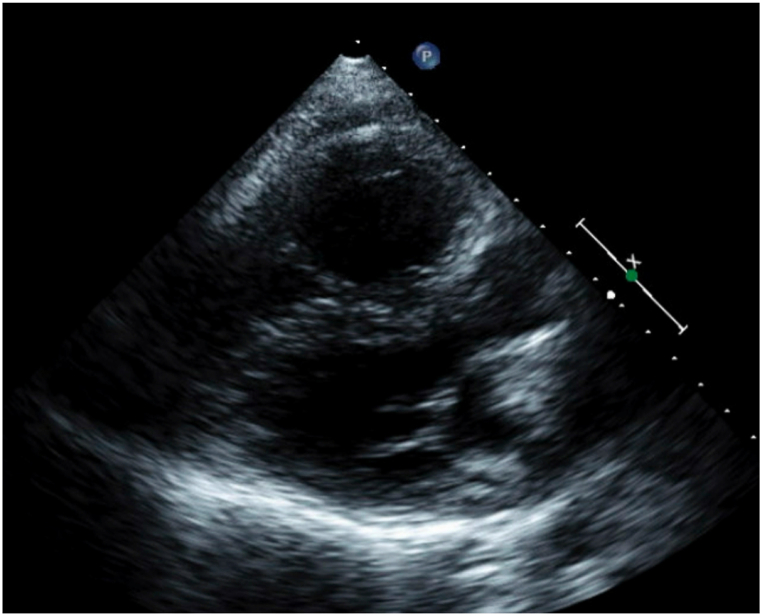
Adult congenital echocardiogram from 2009. Right ventricular enlargement and hypertrophy (RVOT diameter at SAX AV = 31mm; RVIT Apex to basal lateral TV annulus and SAX @ TV = 66 × 41mm), TV DTI SA = 7 cm/s and RVEF = 40 %. Markedly dilated main pulmonary artery (43 mm). RVOT acceleration time = 56 msec consistent with elevated mean pulmonary artery pressure of 54 mmHg.
